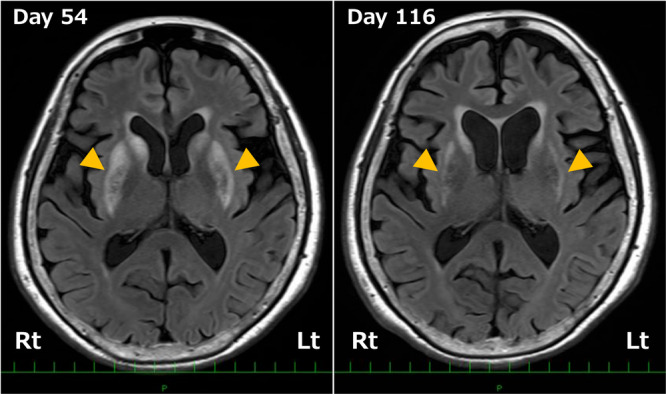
Longitudinal T2‐weighted fluid‐attenuated inversion recovery (FLAIR) axial images of the present case. On Day 54, bilateral hyperintense lesions in the striatum (indicated by the orange triangles) are observed (left image). On Day 116, decreased bilateral striatal hyperintensity and bilateral striatal volume loss (indicated by the orange triangles), with enlargement of the anterior horns of the lateral ventricles, are observed (right image).
